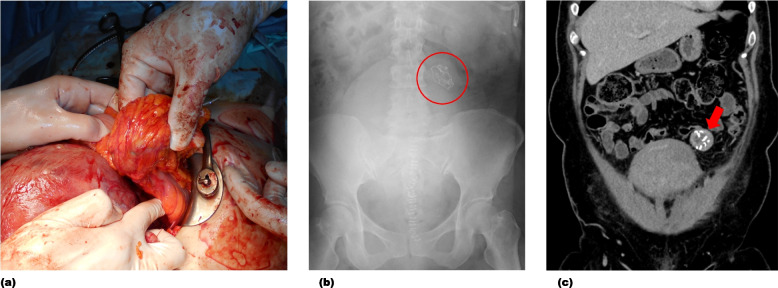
Findings in a patient with a retained surgical item. a A mass, which appeared to be of mesenteric origin and measured approximately 4 cm, was observed above the uterus during a cesarean section performed 2 years after a previous cesarean section. b Postoperative radiographs showing a suspected retained surgical sponge during the current cesarean section. However, because the sponge counts were correct, the current surgery was completed. c Computed tomography performed 2 days after the current cesarean section revealed a retained surgical sponge from the first cesarean section, which required surgical removal
